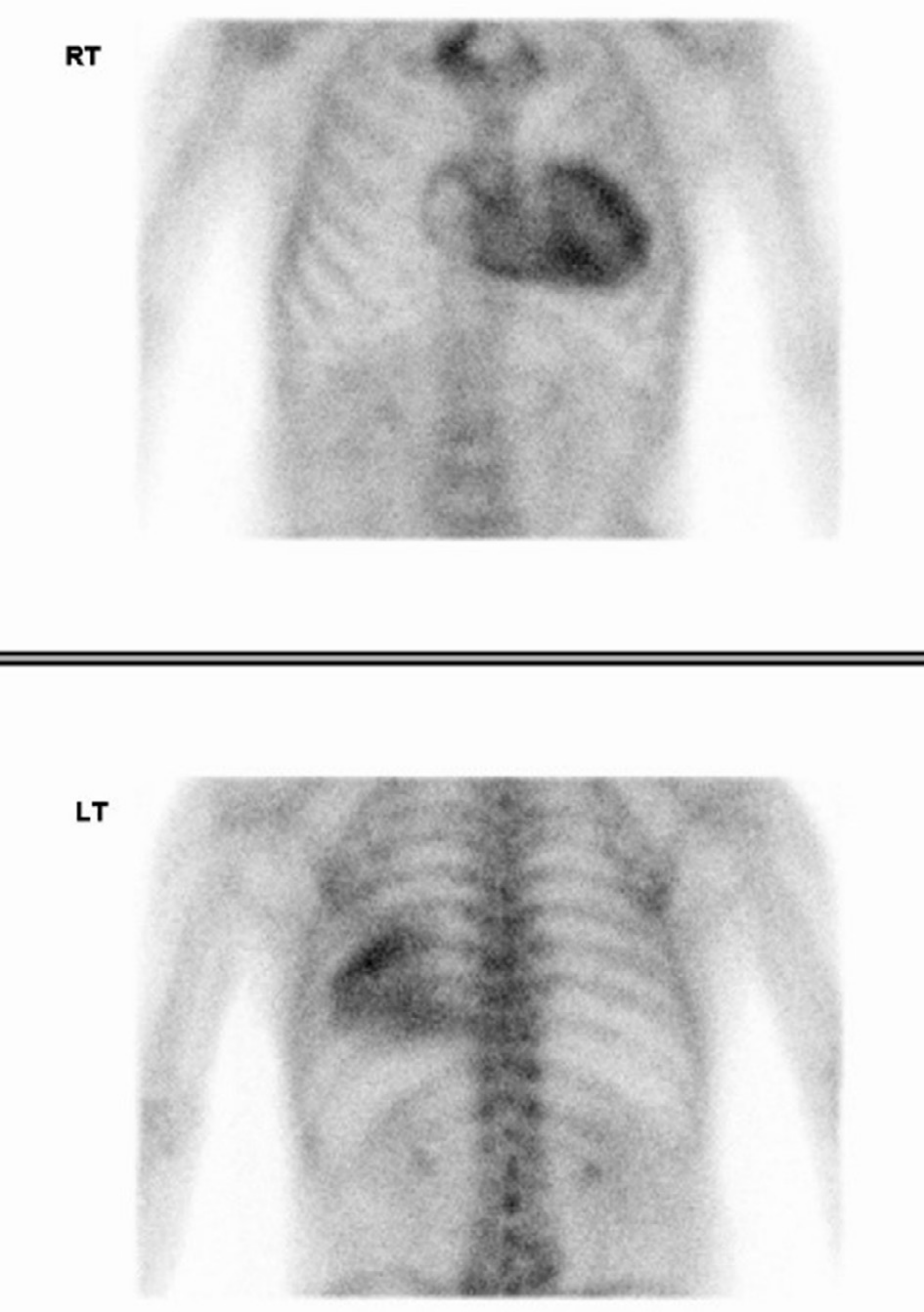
Cardiac scintigraphy in case 2. Anterior and posterior planar thoracic images, acquired 3 h after 99mTc-DPD administration, demonstrate severe myocardial uptake (Perugini 3 – uptake greater than rib uptake) predominantly in the left ventricle, compatible with the diagnosis of cardiac ATTR amyloidosis. Diagnosis was confirmed on endomyocardial biopsy in this case.
